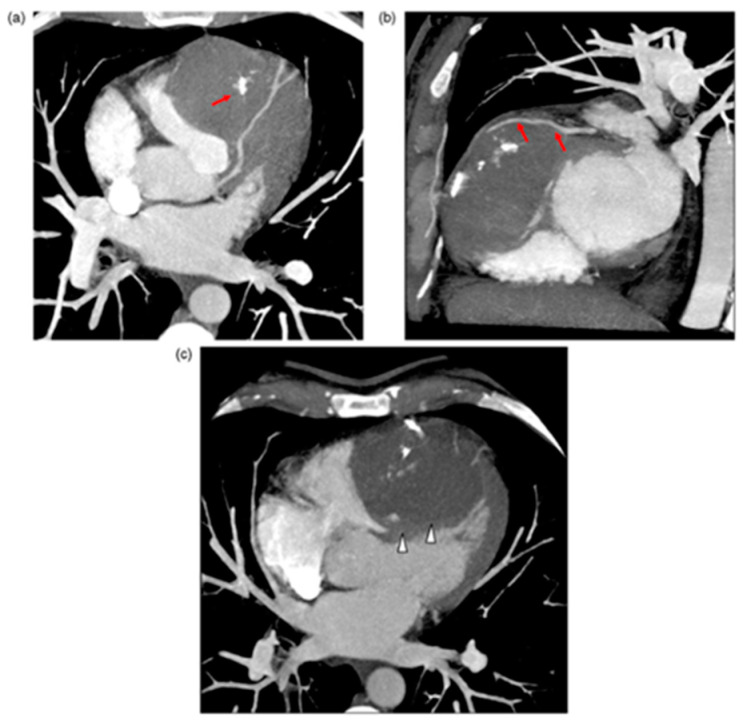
(a) Multi-detector computed tomography (MDCT) axial transverse view. Right ventricular (RV) mass occupying the ventricle and bulging out. The mass is pushing out the RV free wall. Note calcifications in the mass (red arrows). (b) MDCT right oblique short axis view. Left anterior descending artery in contact with fibrous mass tissue (arrows). (c) MDCT with multiplanar reconstruction and Maximum Intensity Pixel on four chamber view. Absence of pericardial effusion. The mass is arising from the IVS (white arrowheads); large sessile adherence on IVS. Stéphant E, et al. Eur. J. Radiol. Extra 2008; 67: e103–e106 [72].
